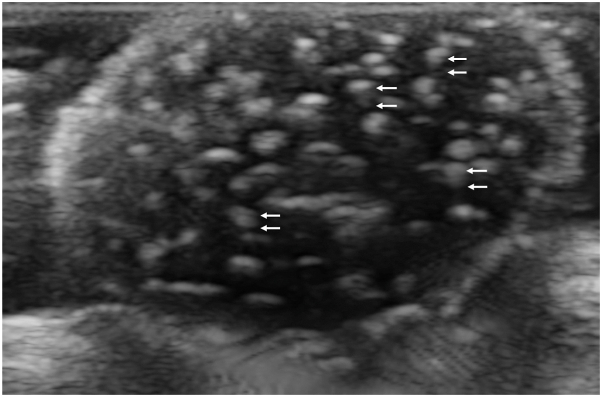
Ultrasonography image showing multiple bright spots with a comet-tail artifact. *White arrows* indicate the start and end points of the comet-tail artifact.
